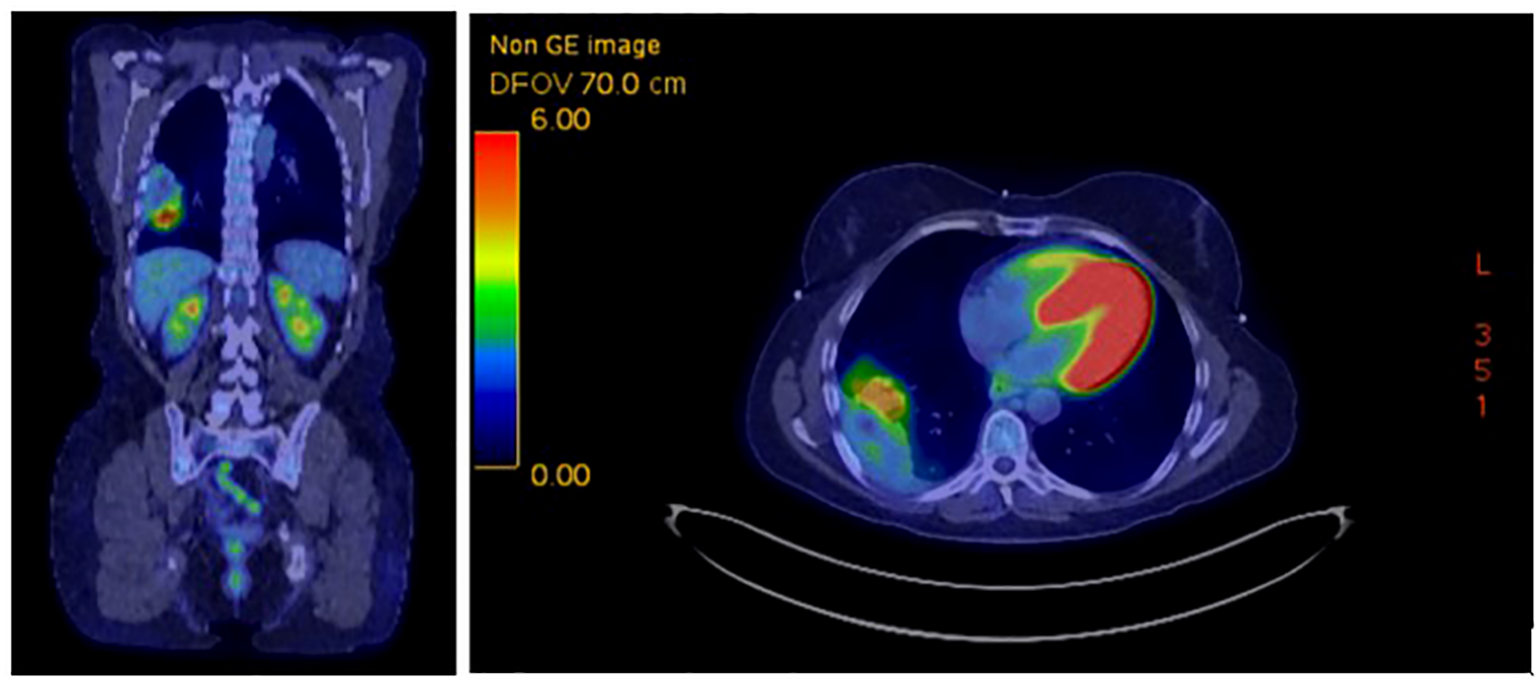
FDG PET 9/22/2022 showing marked response of right lower lobe mass, 6.1 x 3.6 cm with SUV max 6.1.
